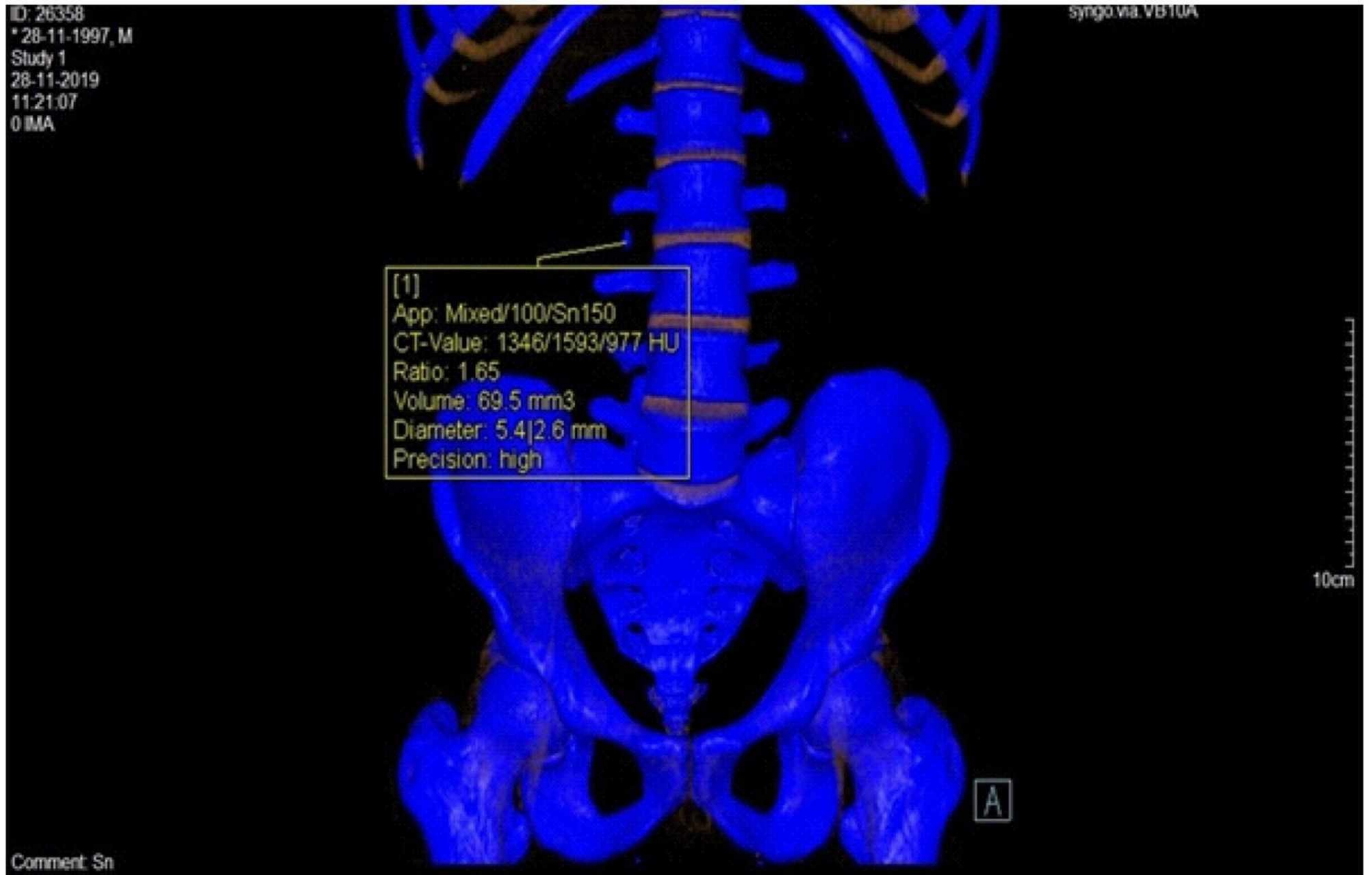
Noncontrast coronal dual-energy CT images with right-sided ureteric calculus CT: computed tomography
